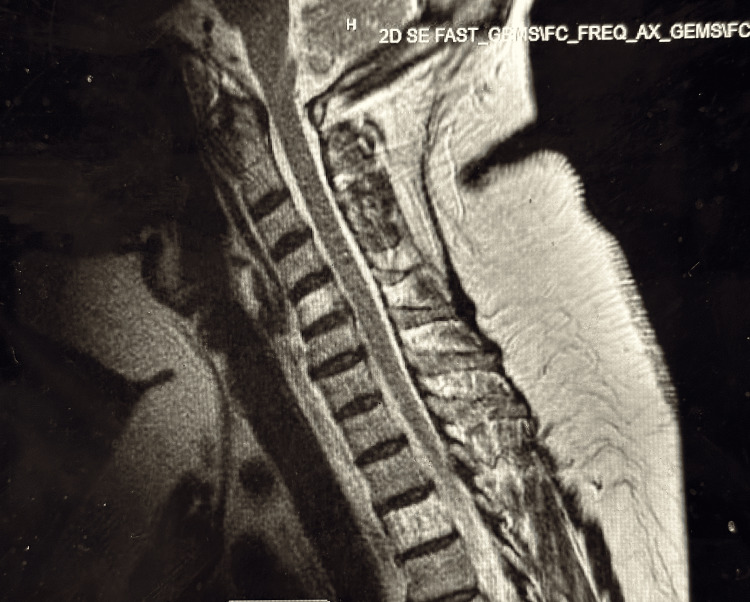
MRI spine cervical There is no evidence of acute abnormality. There is no evidence of spinal cord compression or intrinsic spinal cord abnormality. Mild disc desiccation and disc bulging are present throughout the cervical spine, with no evidence of focal disc herniation or nerve root compression. The cranio-cervical junction is unremarkable. Paraspinal soft tissues do not show any significant abnormality.
